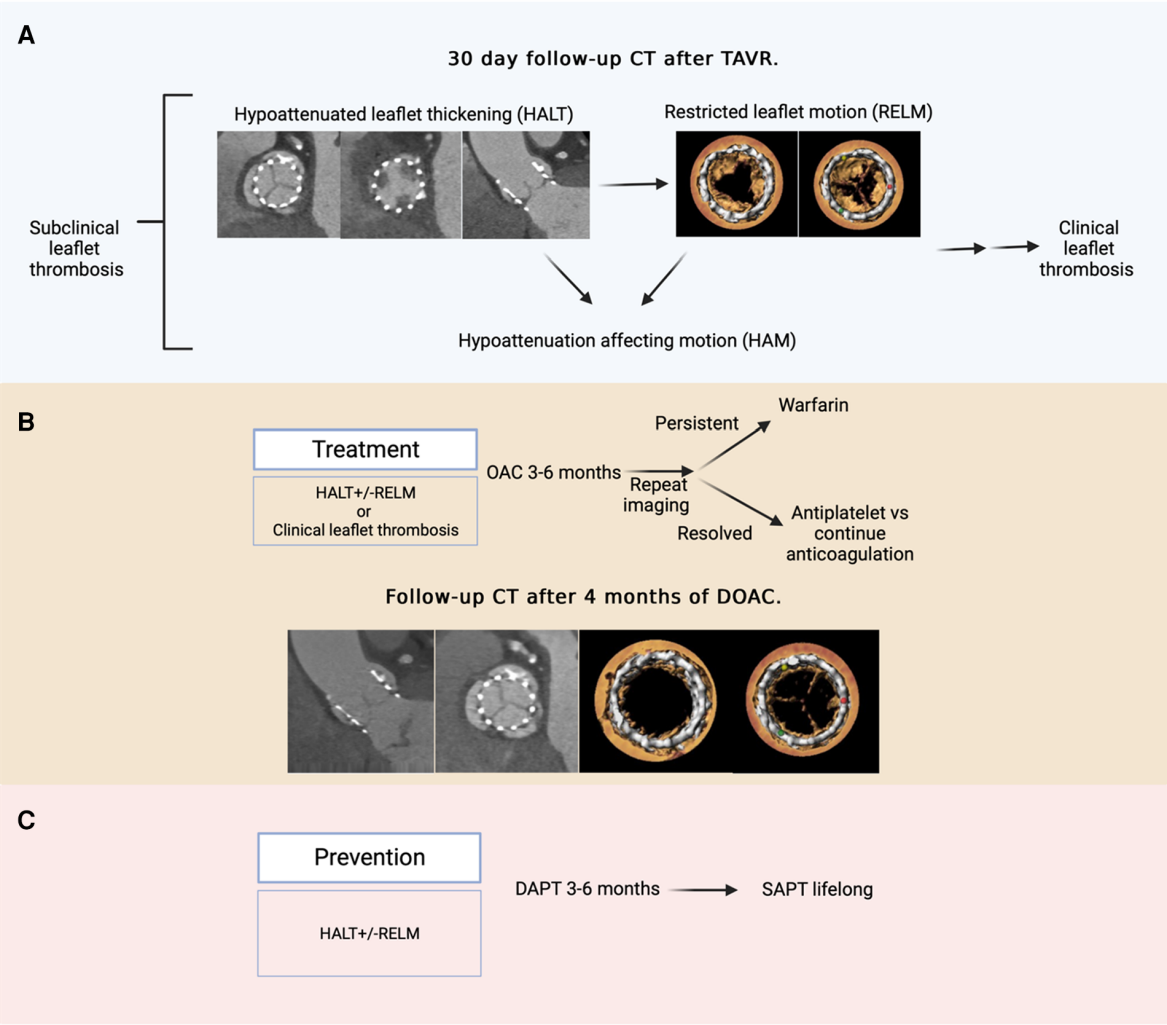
Hypoattenuated leaflet thickening, restricted leaflet motion, and management strategies. (A) An example of a 30-day follow-up CT following TAVR indicating HALT and RELM on all three leaflets and HAM of the non-coronary cusps. If left untreated, SLT may progress to clinical thrombosis, although the absolute risk of this is not yet fully understood. (B) Treatment of SLT involves 3–6 months of oral anticoagulation followed by repeat imaging to determine resolution and subsequent treatment strategy. A follow-up CT of the same patient following 4 months of direct oral anticoagulation (rivaroxaban 10 mg daily) treatment indicated a significant resolution of leaflet thrombosis with HALT observed only on the left coronary cusp and no RELM or HAM. (C) Prevention of SLT involves dual antiplatelet therapy for 3–6 months followed by lifelong single antiplatelet therapy.
